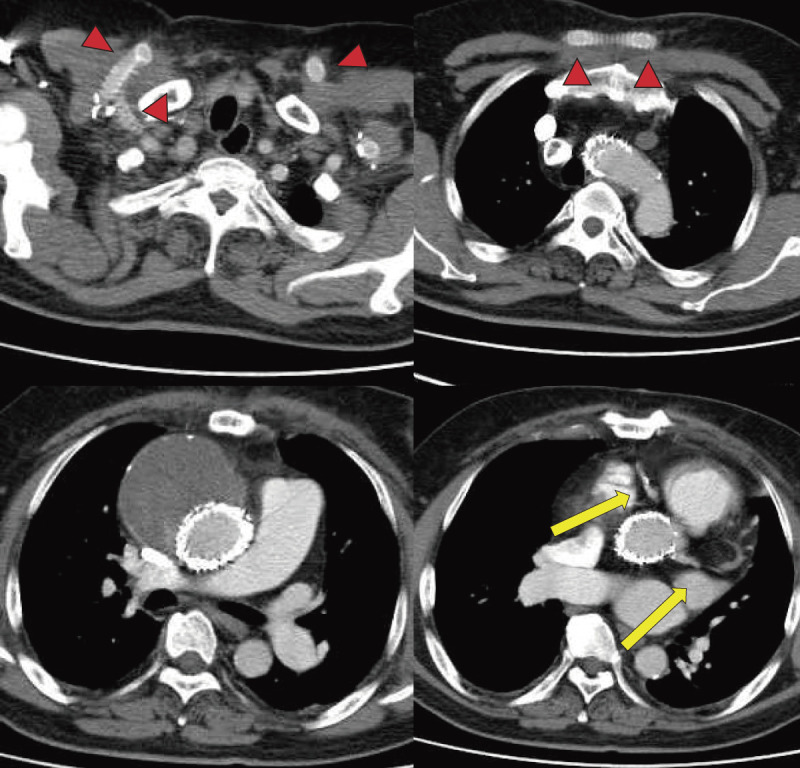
Postoperative CT. The graft remained patent (red arrowheads), and no endoleak was identified. Both right and left coronary arteries were well opacified, confirming preserved coronary flow (yellow arrows). CT: computed tomography
